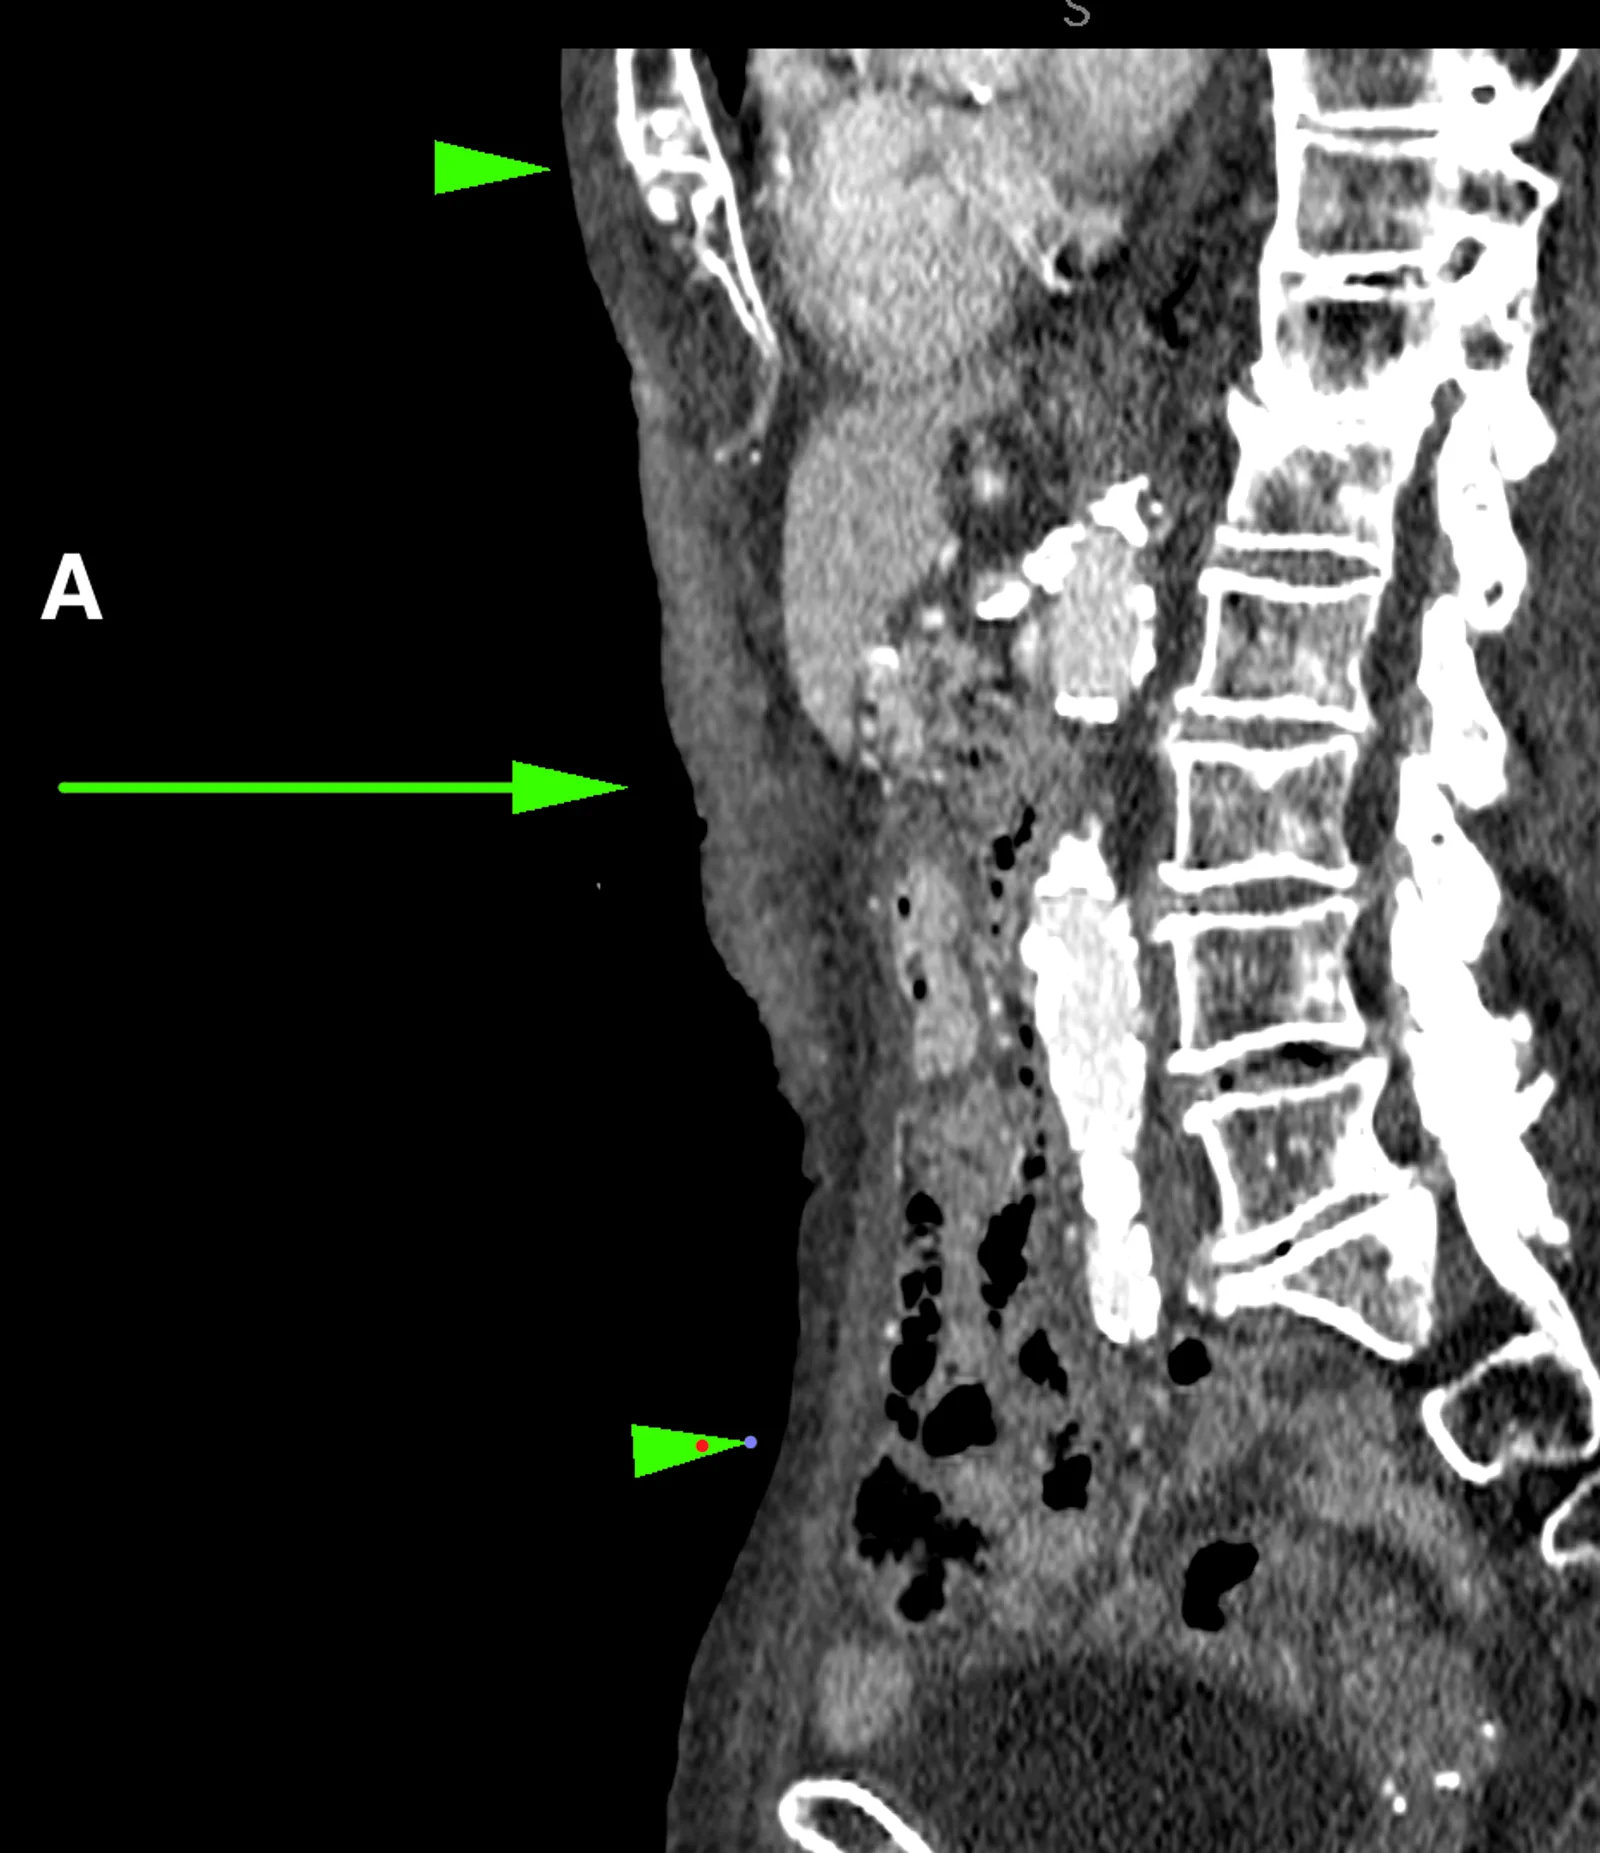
Contrast-enhanced CT scan demonstrating further irregular skin thickening of the abdomen (green arrow). The arrowheads point to areas of no skin thickening. A, anterior of the patient; CT, computed tomography
